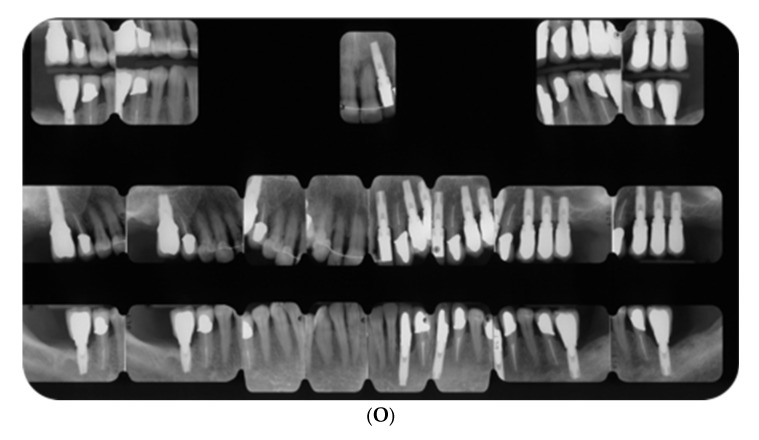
A fifty-two-year-old female with generalized Stage IV periodontitis, PTM of tooth 12 in occlusal trauma. (A–C): Baseline clinical view. (D): Baseline full-mouth radiographs. (E–I): Fixed OT following active periodontal therapy. First, minor levelling and space opening for proper alignment of tooth 12 were obtained. Thereafter, tooth 12 was intruded according to the segmented arch technique, using an implant anchorage on tooth 16 and a cantilever activated with light forces of 10 g. Treatment lasted 10 months, thereafter the patient was referred for final prosthetic rehabilitation. (J,K): Periapical radiographs of tooth 12 at baseline and at T3. (L–O): Clinical view and full-mouth radiographs after 12 years of SPC. Implant-supported restorations were placed to replace missing or extracted teeth during the initial phase of periodontal treatment. No additional teeth were lost.
